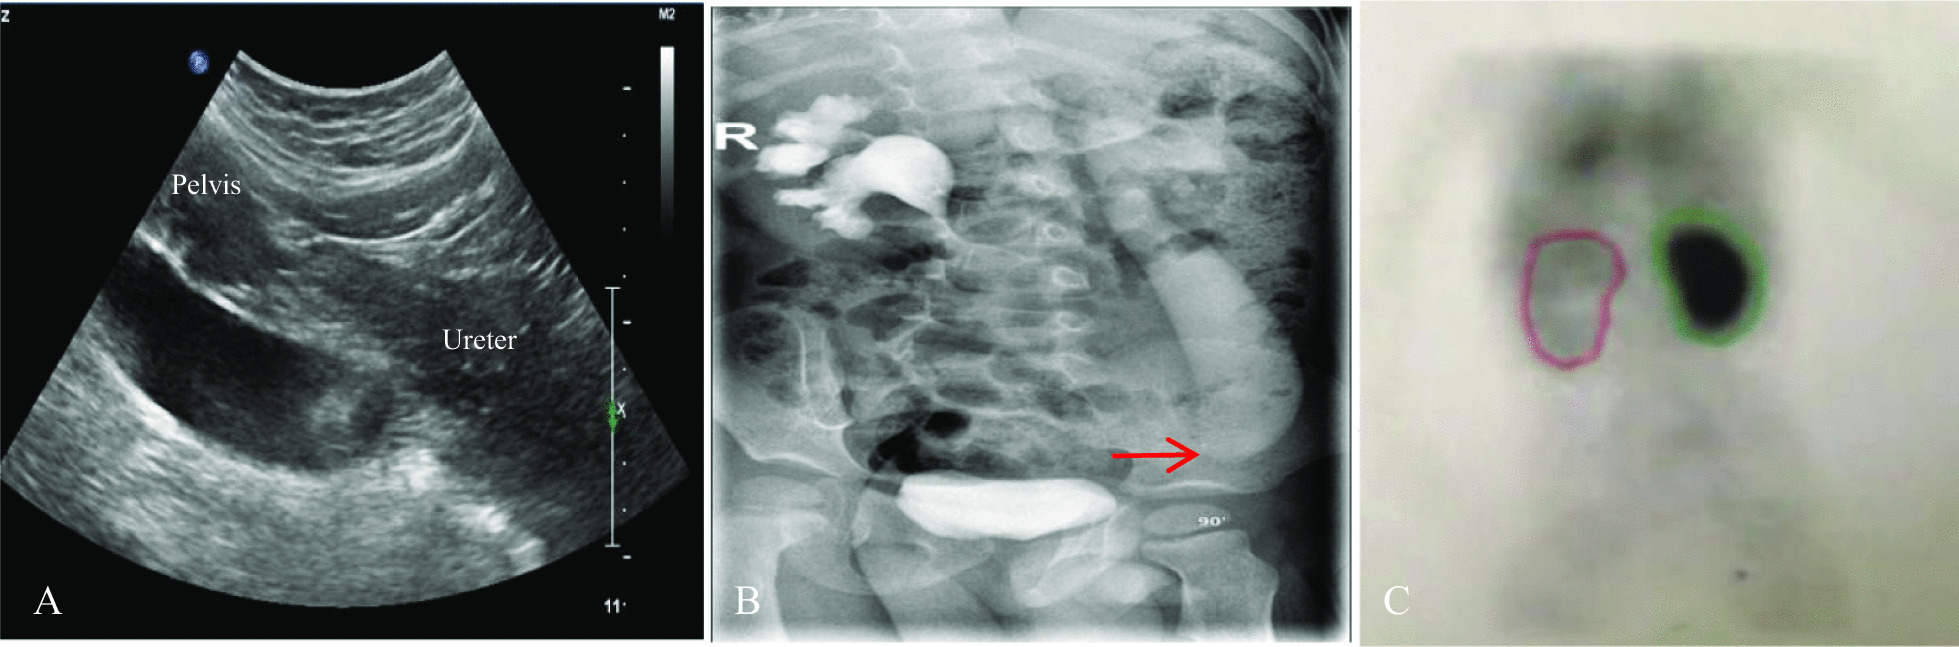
A 1.2-year-old boy. a Ultrasound of the affected kidney shows hydronephrosis and hydroureter. b IVU reveals the left midureteral stenosis (arrow); c preoperative DTPA renography demonstrated delayed drainage of the left kidney
